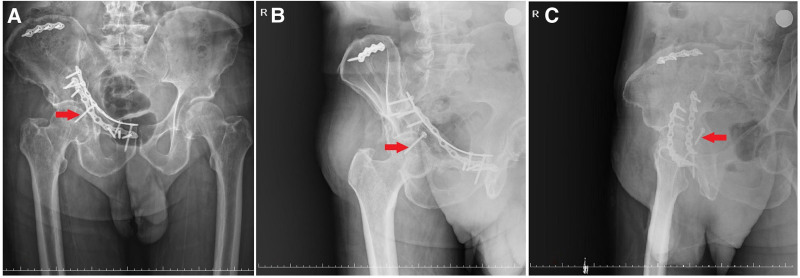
(A) Postoperative anteroposterior pelvic X-ray image. (B) Postoperative obturator oblique pelvic X-ray image. (C) Postoperative iliac oblique pelvic X-ray image (red arrows: posterior wall lag screw).
